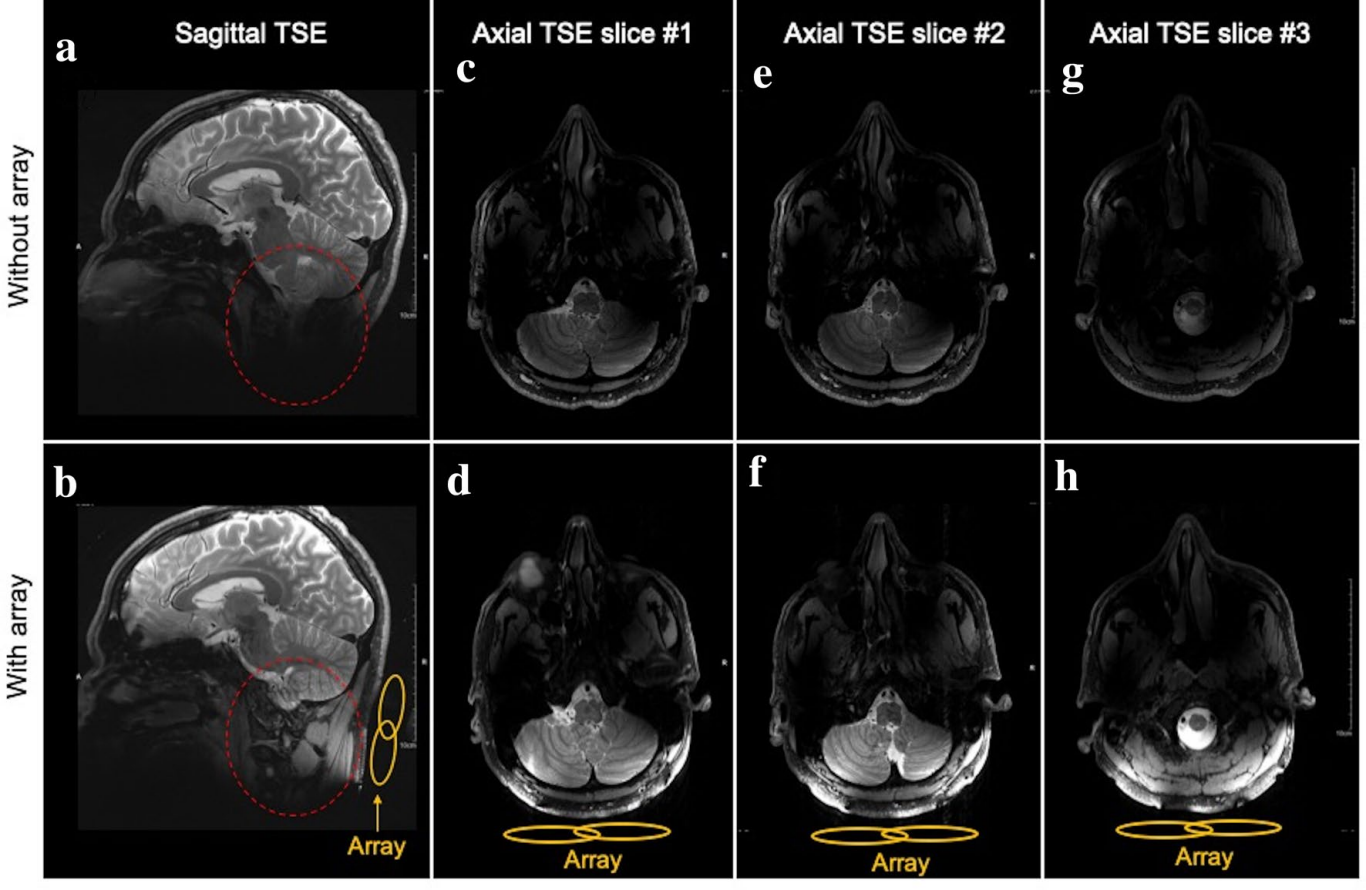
In vivo brain MRI at 7T. Sagittal T2-weighted TSE images obtained without (a) and with (b) the RF array show significant SNR and CNR improvement in the inferior regions in the presence of the array. In particular, the cerebellum, brainstem, and neck muscle are more clearly visible using the RF array. Axial T2-Weighted TSE slices obtained at various locations in the lower brain. Images obtained in slice #1 (c,d) show that placing the RF array results in significant improvement in $${B}_{1}^{+}$$ uniformity and SNR in the cerebellum and brainstem. Slice #2 (e,f) and slice #3 (g,h) obtained at the physical border and 2 cm extended outside of the border, respectively. These images show that using the RF array extends the anatomical coverage of the head coil visualizing vertebral artery and spinal cord, which are hardly visible without the array.
